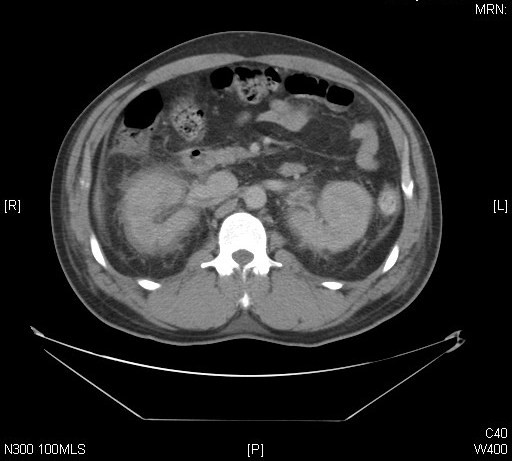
Computed tomography of abdomen on presentation.
